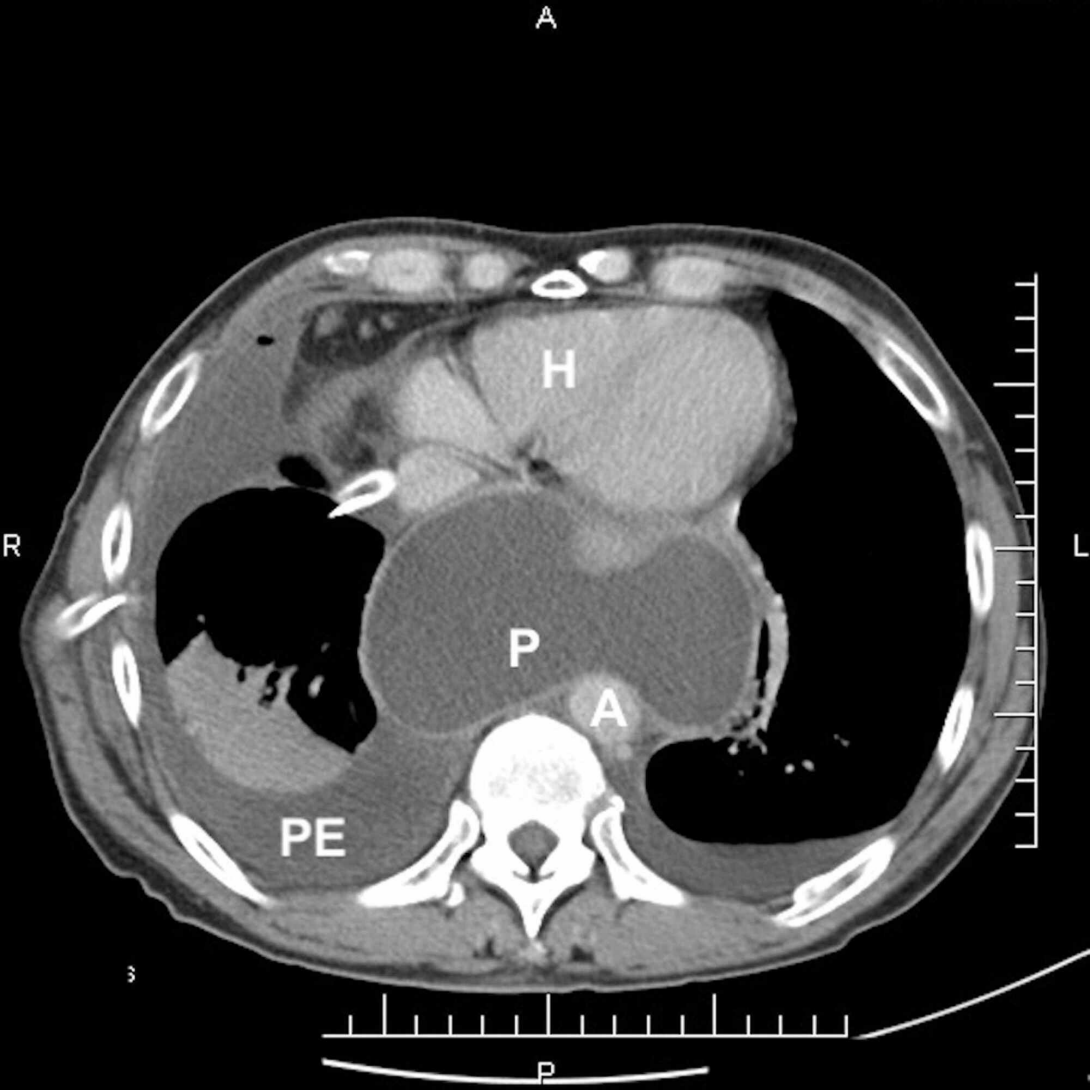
Chest CT scan (axial plane) at the level of the lower mediastinum reveals a mass (P- pseudocyst) and right sided pleural effusion (PE). Heart (H), aorta (A).
